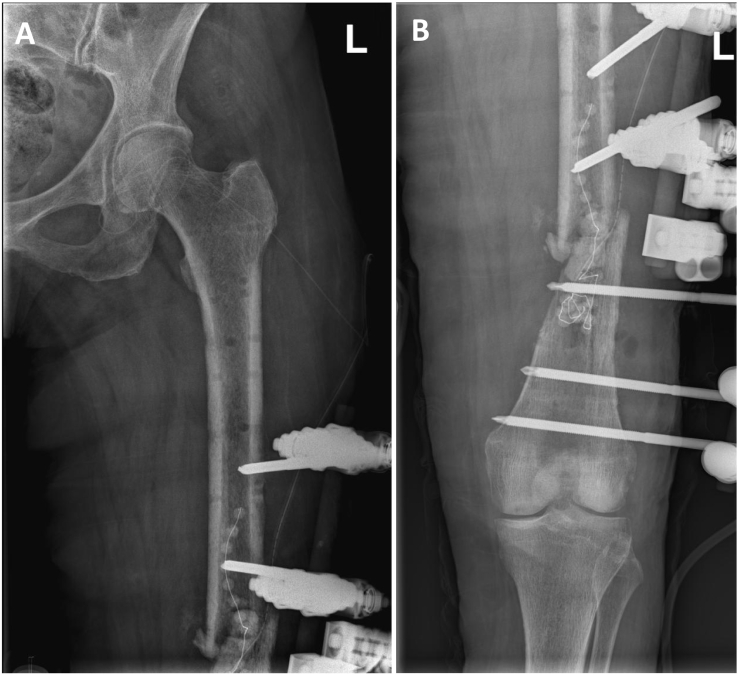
Postoperative X-rays after removal of the external hybrid fixation, debridement of the infected non-union site, placement of antibiotic beads and re-osteosynthesis by external fixation.
